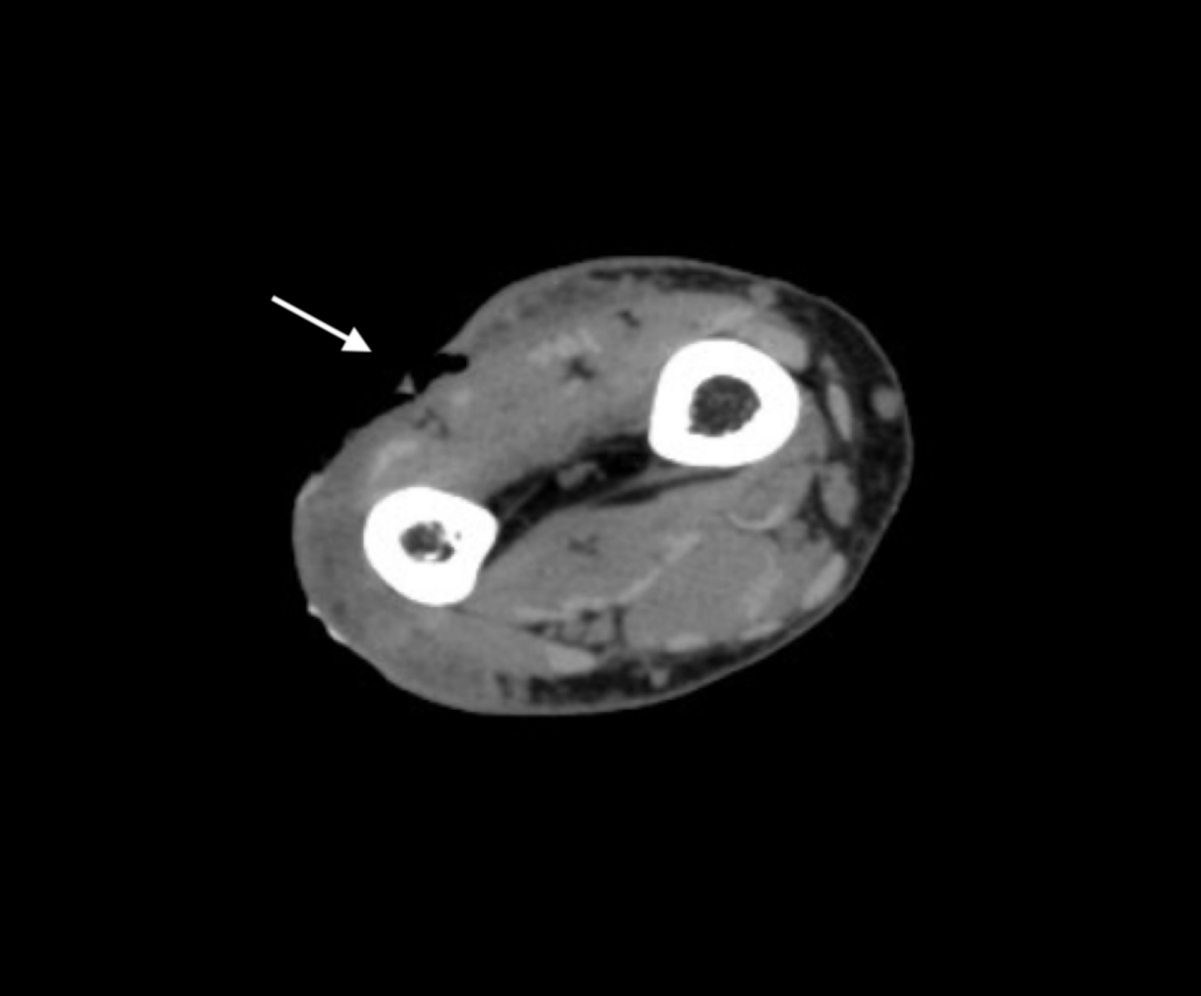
CT scan (axial view) of the right arm at the presentation Limited superficial aerial images (arrow) showing inhomogeneity of the subcutaneous fat.
